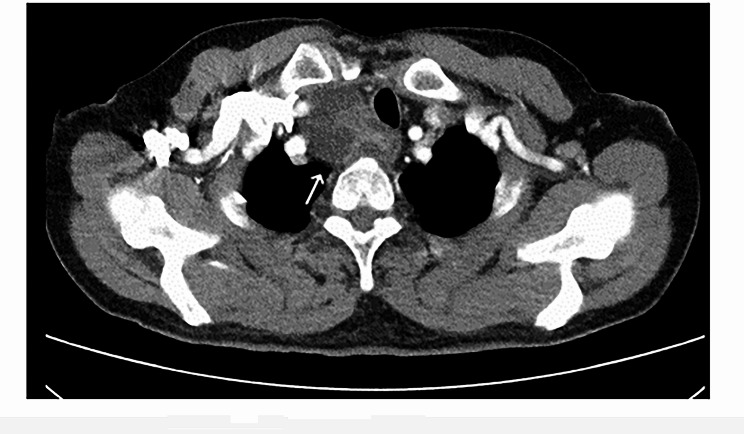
CE-CT demonstrated compression and displacement of the trachea and esophagus to the left by the nodule in the right thyroid lobe
